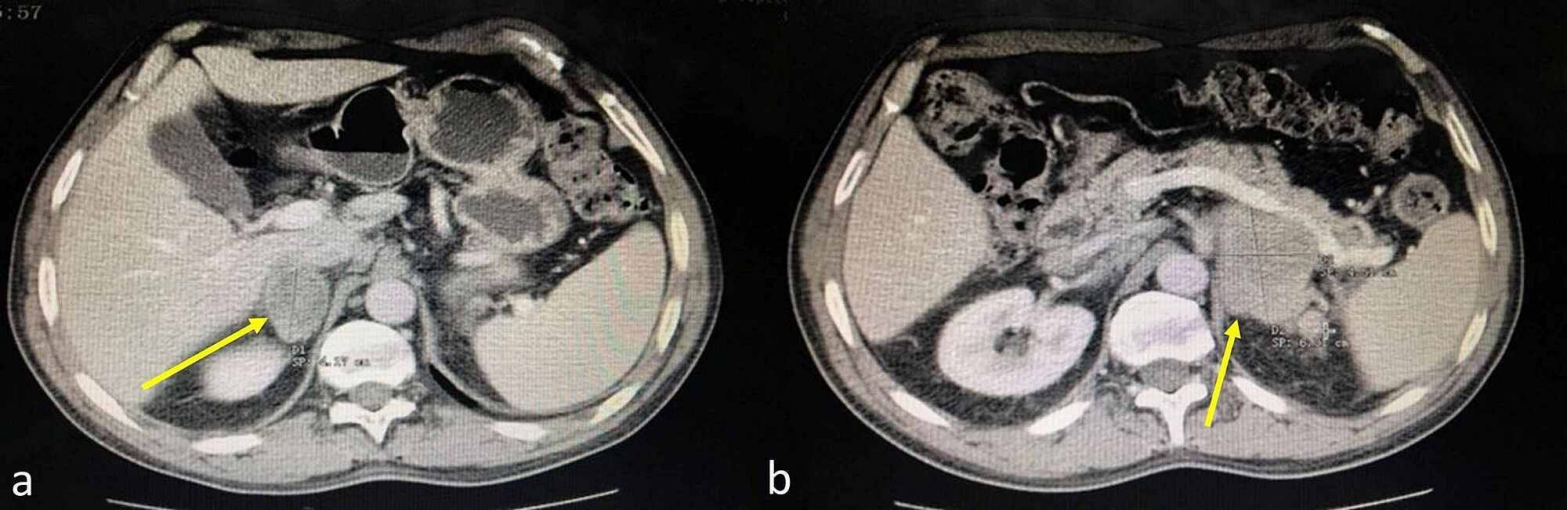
Masses of adrenal glands on thoracoabdominopelvic CT (arrows) CT: computed tomography
